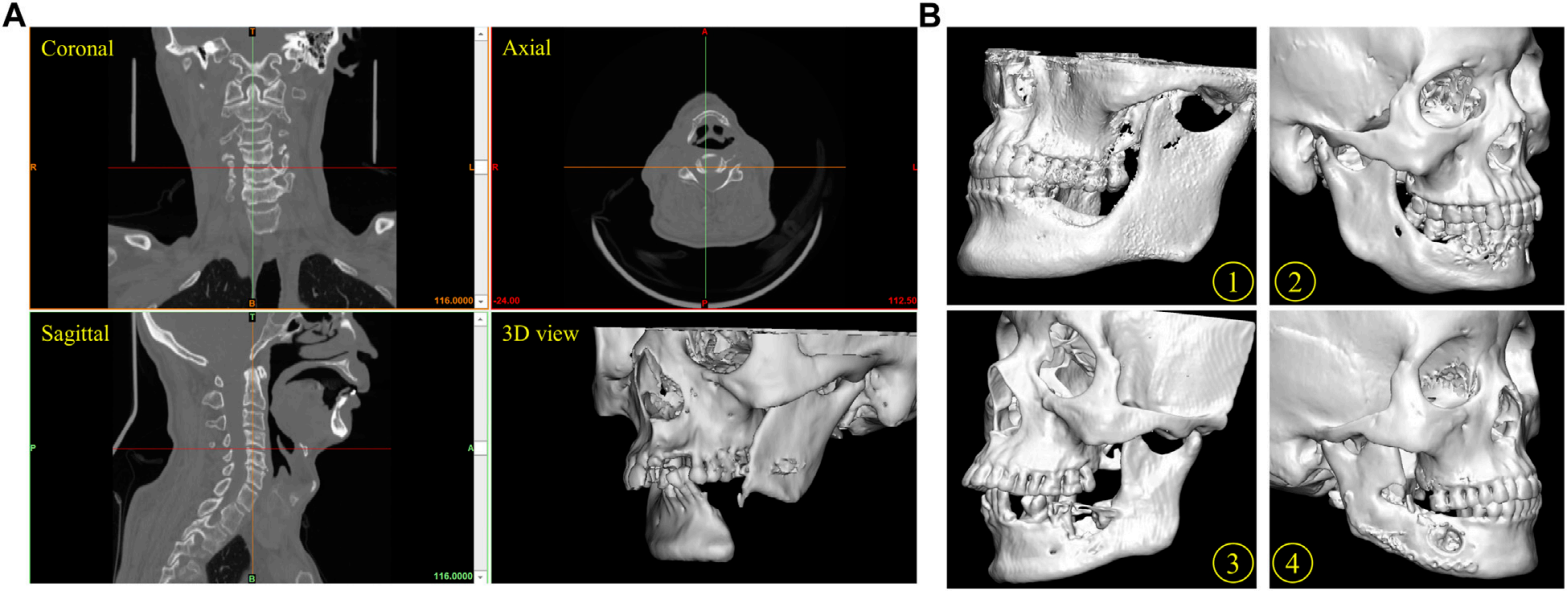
Reconstruction of mandible defect based on CBCT image. (A) The process for the generation of a three-dimensional (3D) mandibular reconstruction. Coronal view, axial view, sagittal view and 3D model of mandibular. (B) Several mandibular defects data were used in this study. Patients 1, 2, 3 and 4.
